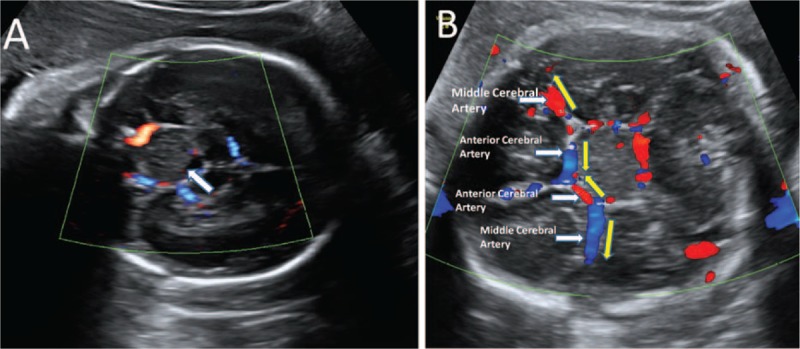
Fetal ultrasonography performed in the 30th week of gestation showed a 2.5 × 2.3 × 2.1 cm^3^ slightly heterogeneous solid mass with a little cystic component (arrowhead) in the sellar region (A) with peripheral encirclement by arterial circle of Willis (B). The red and blue colors indicate the direction of blood flow (yellow arrowhead).
